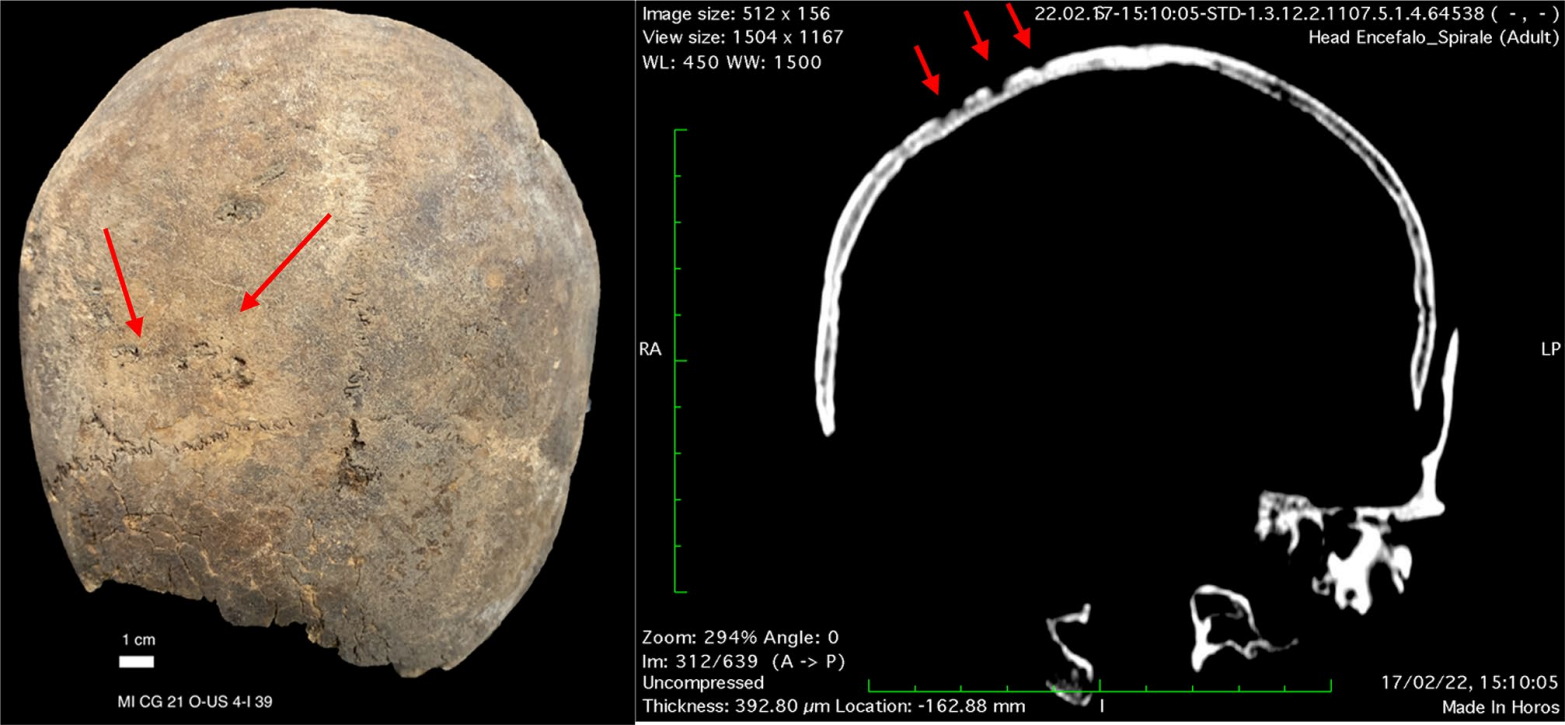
Signs of tertiary syphilis on cranium MI CG 21 O-US 4-I 39 (red arrow), both photographs and CT image.
